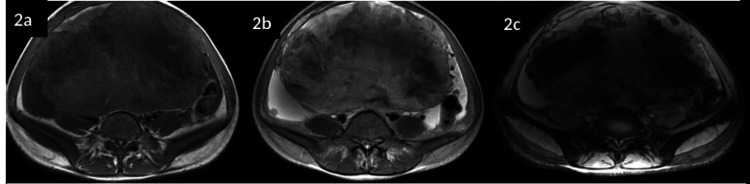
Preoperative MRI signals suggesting possibility of malignancy a: Mass displays an intermediate signal with areas of high signal on T1; b: Inhomogeneous high signal on T2; c: Multiple foci and areas of blooming are seen within the mass on gradient echo (GRE)
